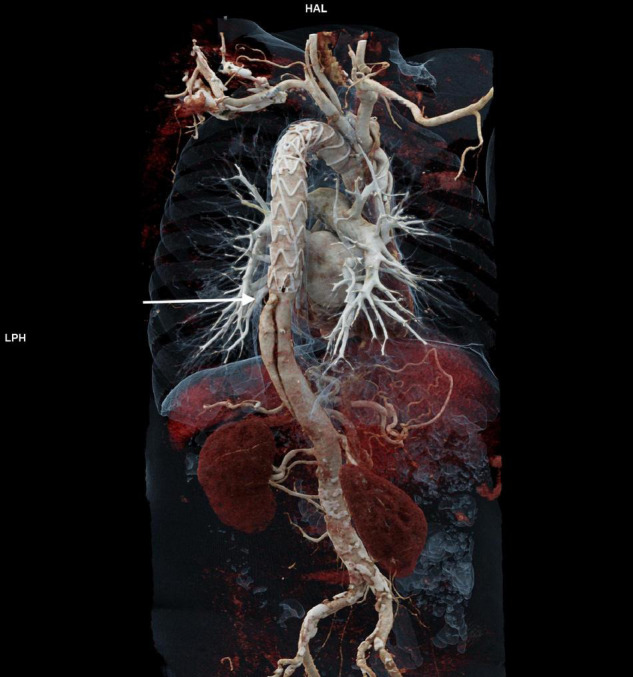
Shows a three dimensional CTA reconstruction of a patient with dSINE (arrow) which causes recurrent false lumen perfusion.
